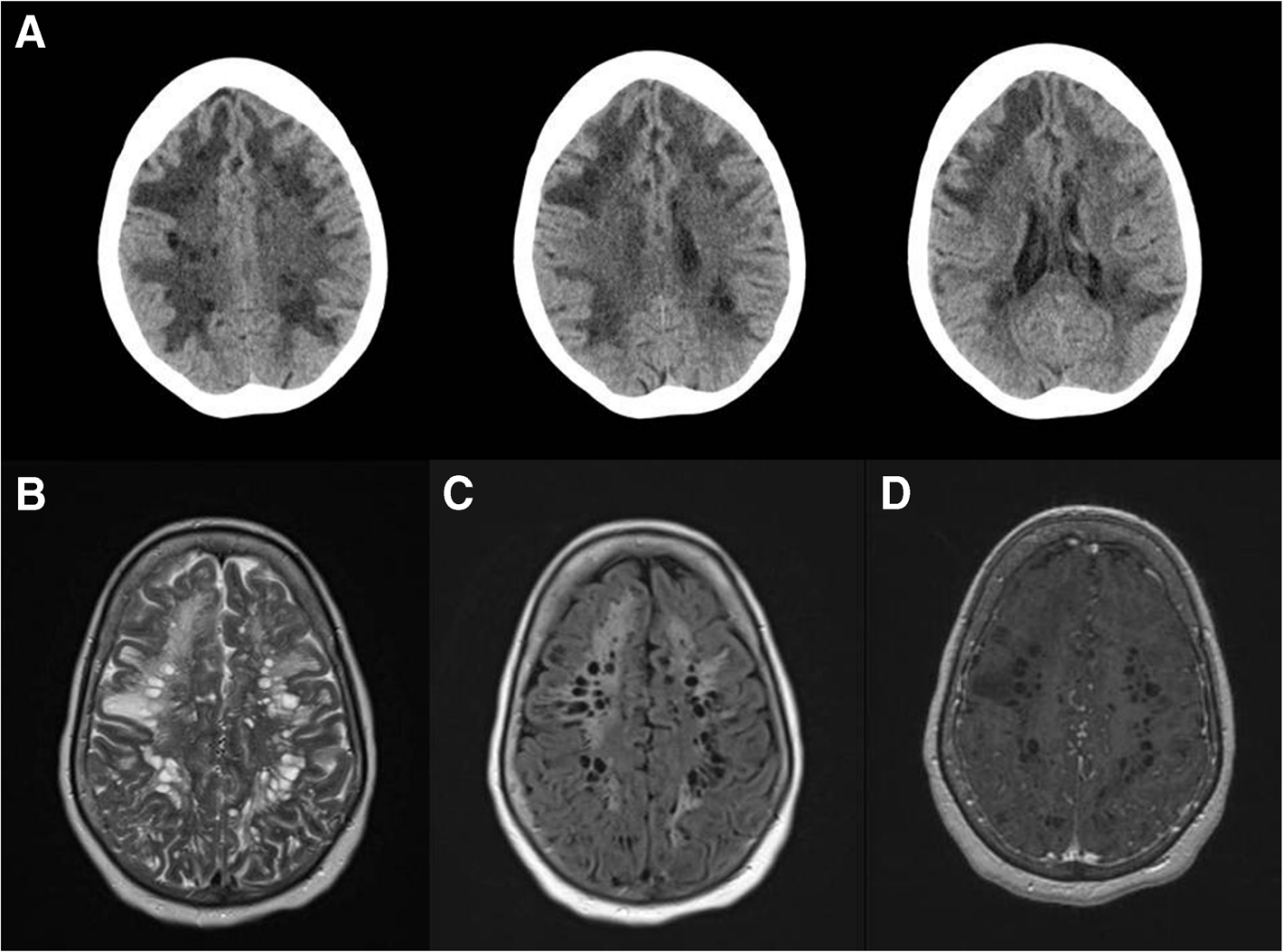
Computed tomography and magnetic resonance imaging of the brain in the axial plane. (A) Non-enhanced computed tomography images of the head showing diffuse leukoencephalopathy involving the frontal, parietal and temporal lobes with relative sparing of the cortex. Brain magnetic resonance imaging showed extensive white-matter abnormality throughout both hemispheres, greatest in the superior convexities, consisting of numerous collections of cystic spaces on T2-weighted (B) and fluid-attenuated inversion recovery (C) images. The spaces have smooth margins and are roughly elongated parallel to the expected direction of the vascular and radial fibers, suggestive of tumefactive perivascular spaces. Many of the spaces have adjacent T2-weighted and fluid-attenuated inversion recovery parenchymal hyperintensity. There was no enhancement after gadolinium contrast administration (D).
